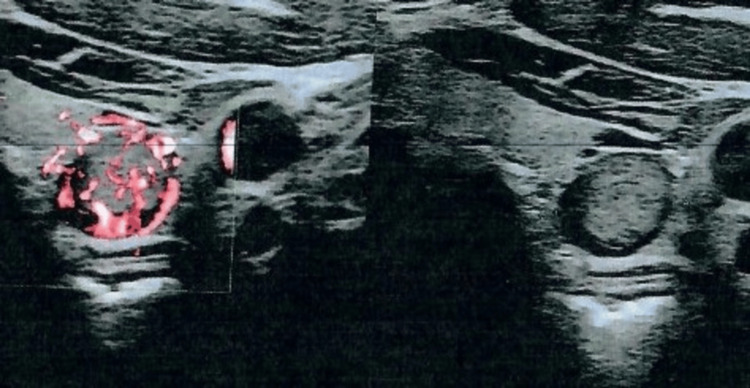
Ultrasound showing a hypoechoic lesion of 16x13.2x8 mm between the mid-portion and the inferior pole of the left thyroid lobe
